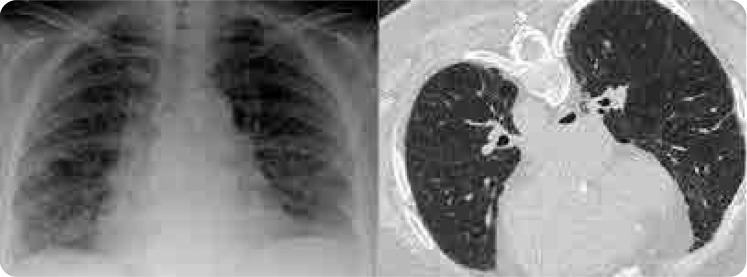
Patient's X-ray and HRCT images after MTX discontinuation: Chest X-ray and HRCT showed significant improvement.
